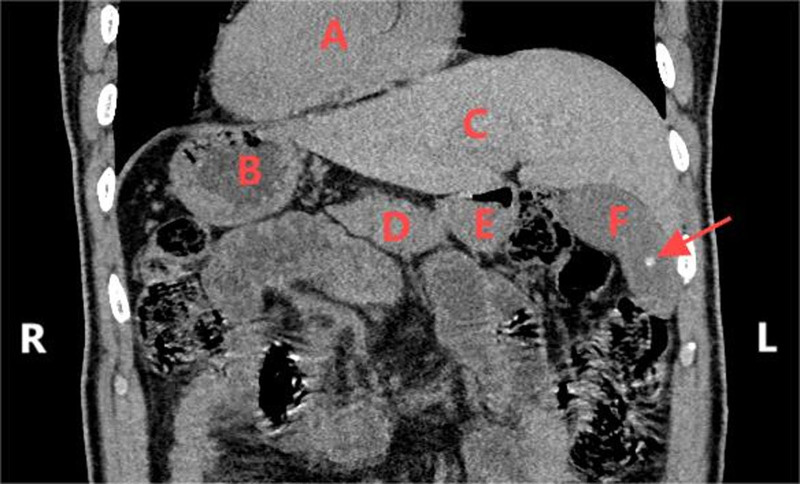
Computed tomography scan results upon admission.
